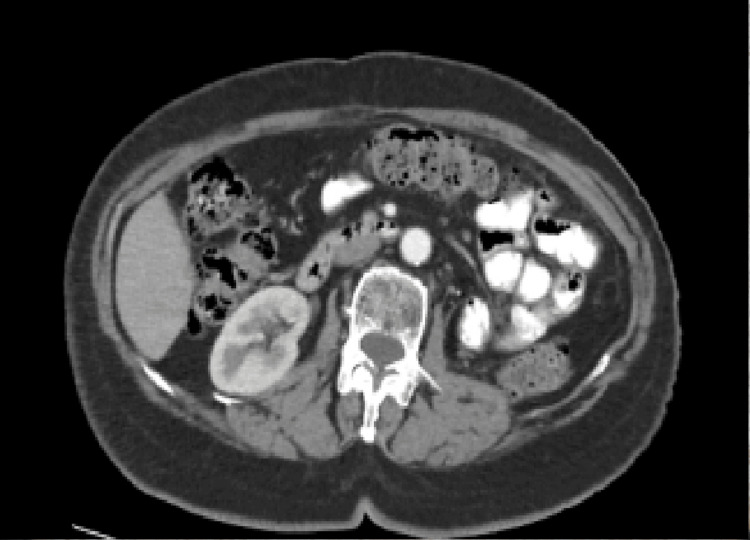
CT scan with IV contrast at six months after operation Six months after surgery, the CT scan was normal, and no site of recurrence was observed.
